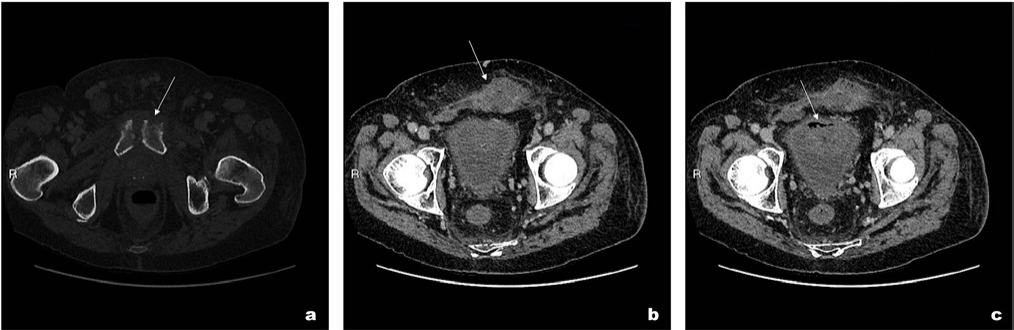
(a) CT axial (bone window) slice demonstrates irregular cortical bony destruction at the pubic symphysis with surrounding free fluid in keeping with osteomyelitis. (b) Axial slice (soft tissue window) in the portal venous phase demonstrate fluid collection within the anterior abdominal wall. (c) Thick-walled bladder with intraluminal air seen in the same window as Figure 1.
